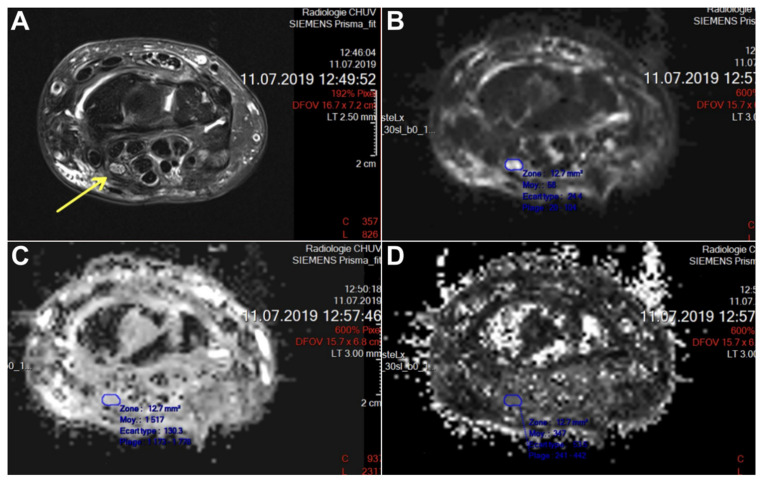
(A) The identification of the median nerve (arrow) on the T1-weighted sequence; (B) encircling the median nerve on the DWI trace sequence; (C) the encircled region of interest copied on the ADC sequence (D) and on the FA sequence.
